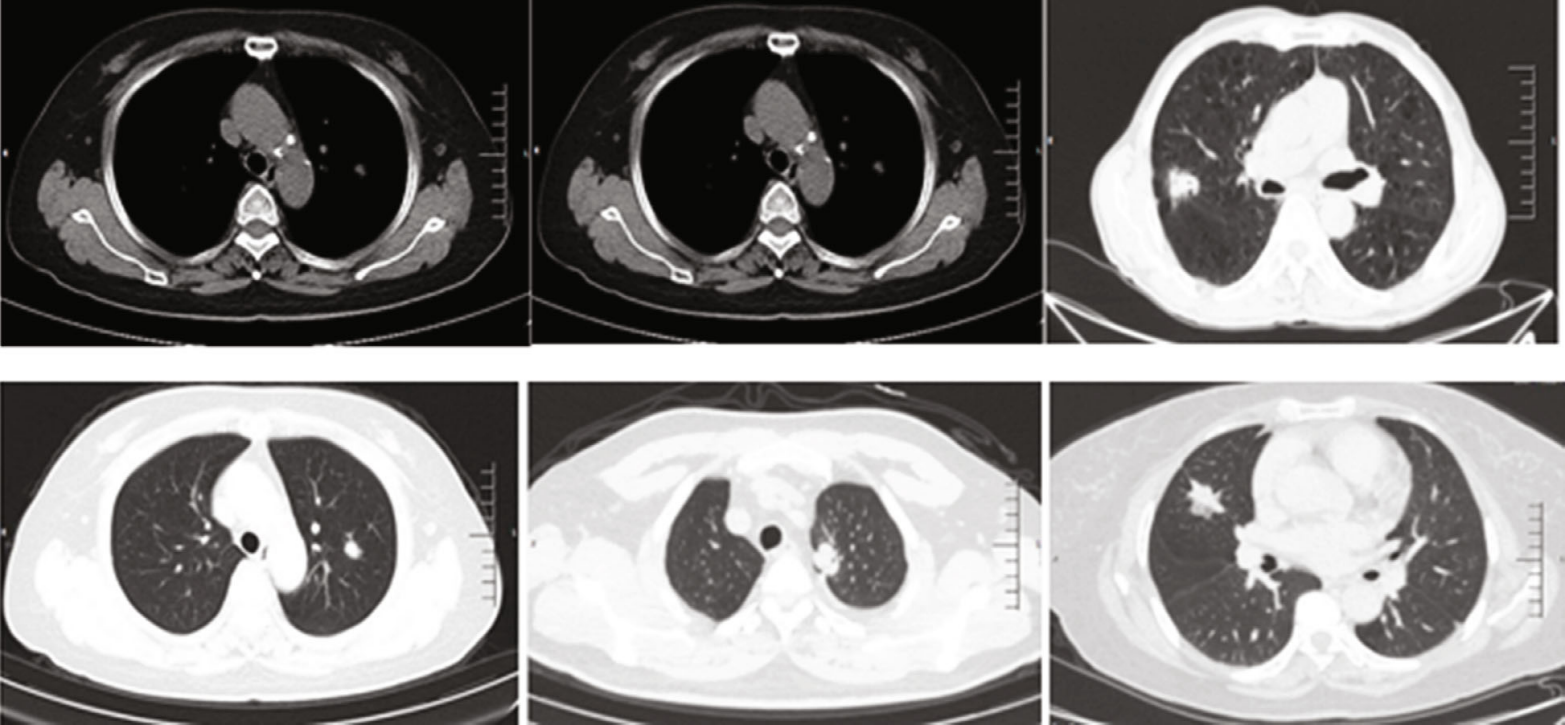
CT image samples of pulmonary nodules.
